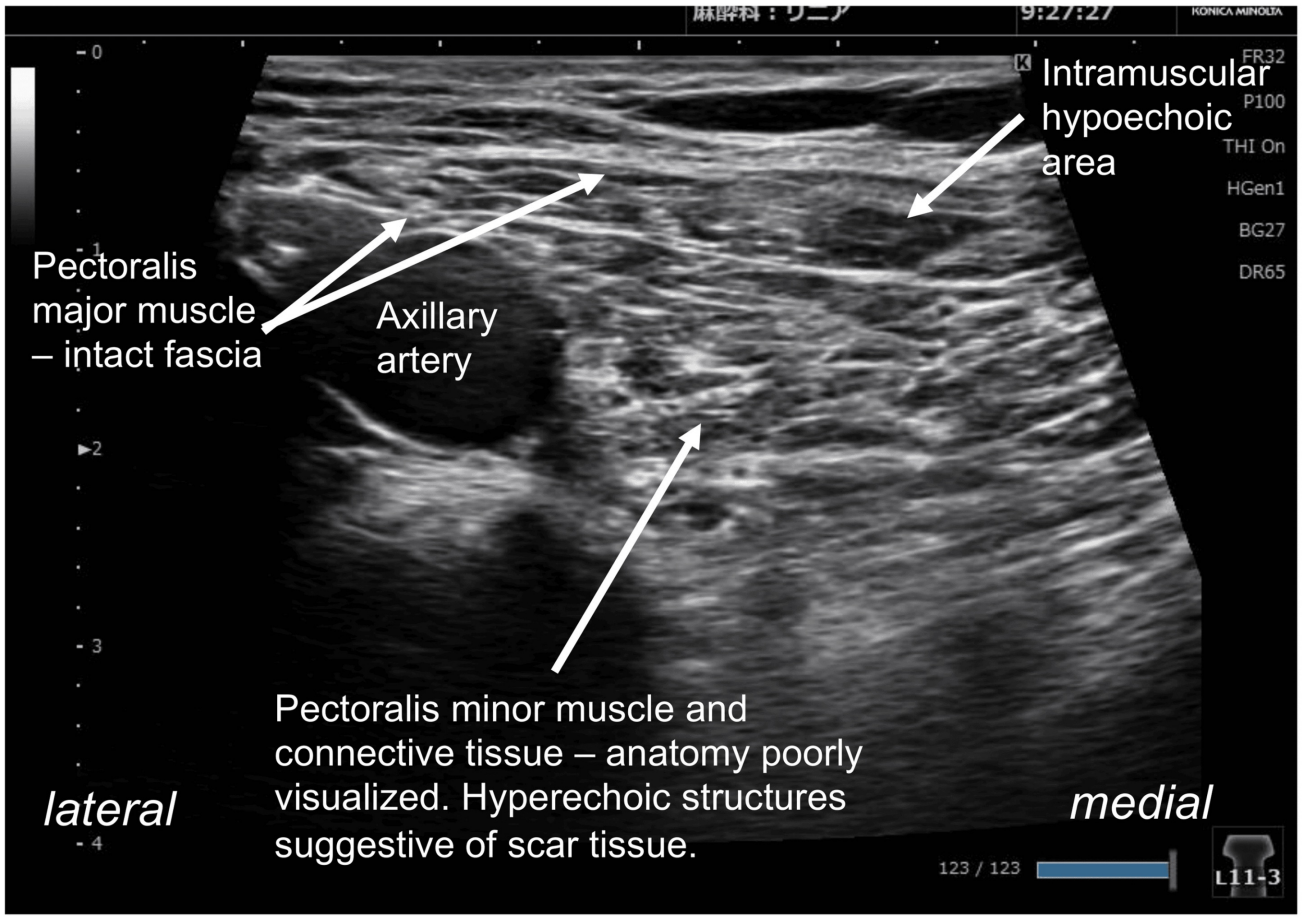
Ultrasonographic findings near the right axillary artery Ultrasound image showing a mixture of hyperechoic and hypoechoic regions around the right axillary artery. These findings indicate localized structural abnormalities in the adjacent pectoralis major and minor muscles.
